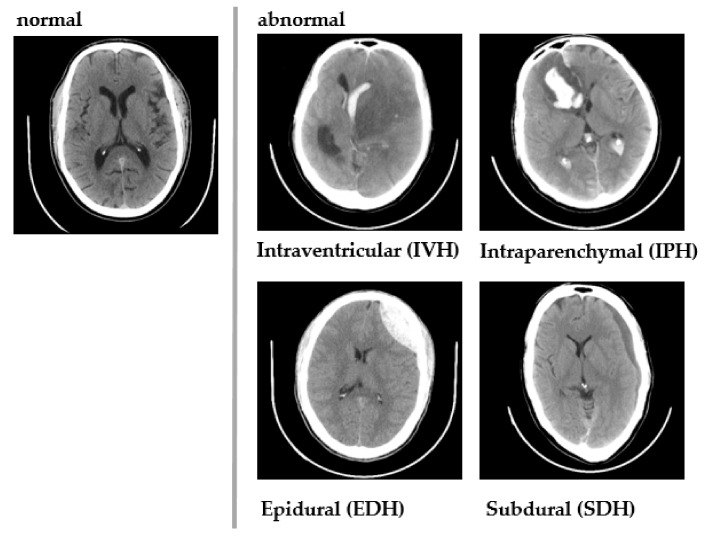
Sample CT images from CQ500 dataset.
